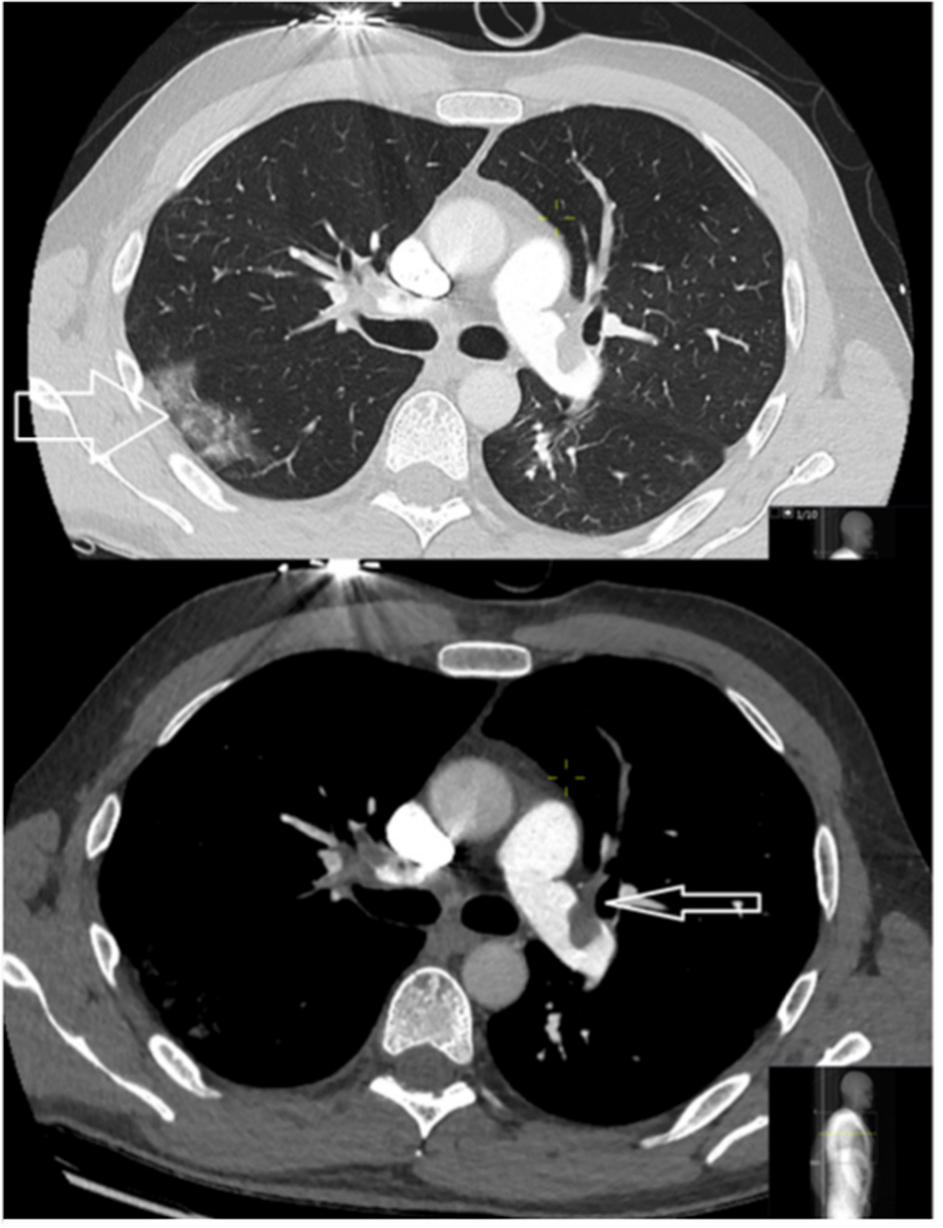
CT images of two different patients with COVID-19. A middle-aged male with limited peripheral ground glass opacities (large arrow) and large central emboli (small arrow).
